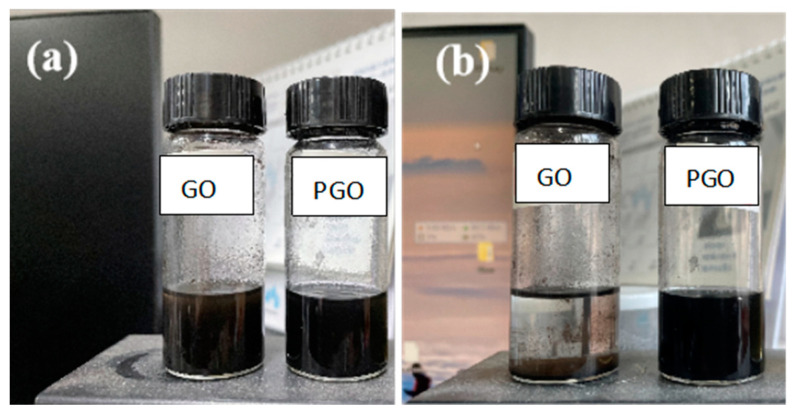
Digital photos of dispersion of GO and PGO in n-butanol: (a) just after ultrasonic irradiation and (b) after 15 min of standing.
